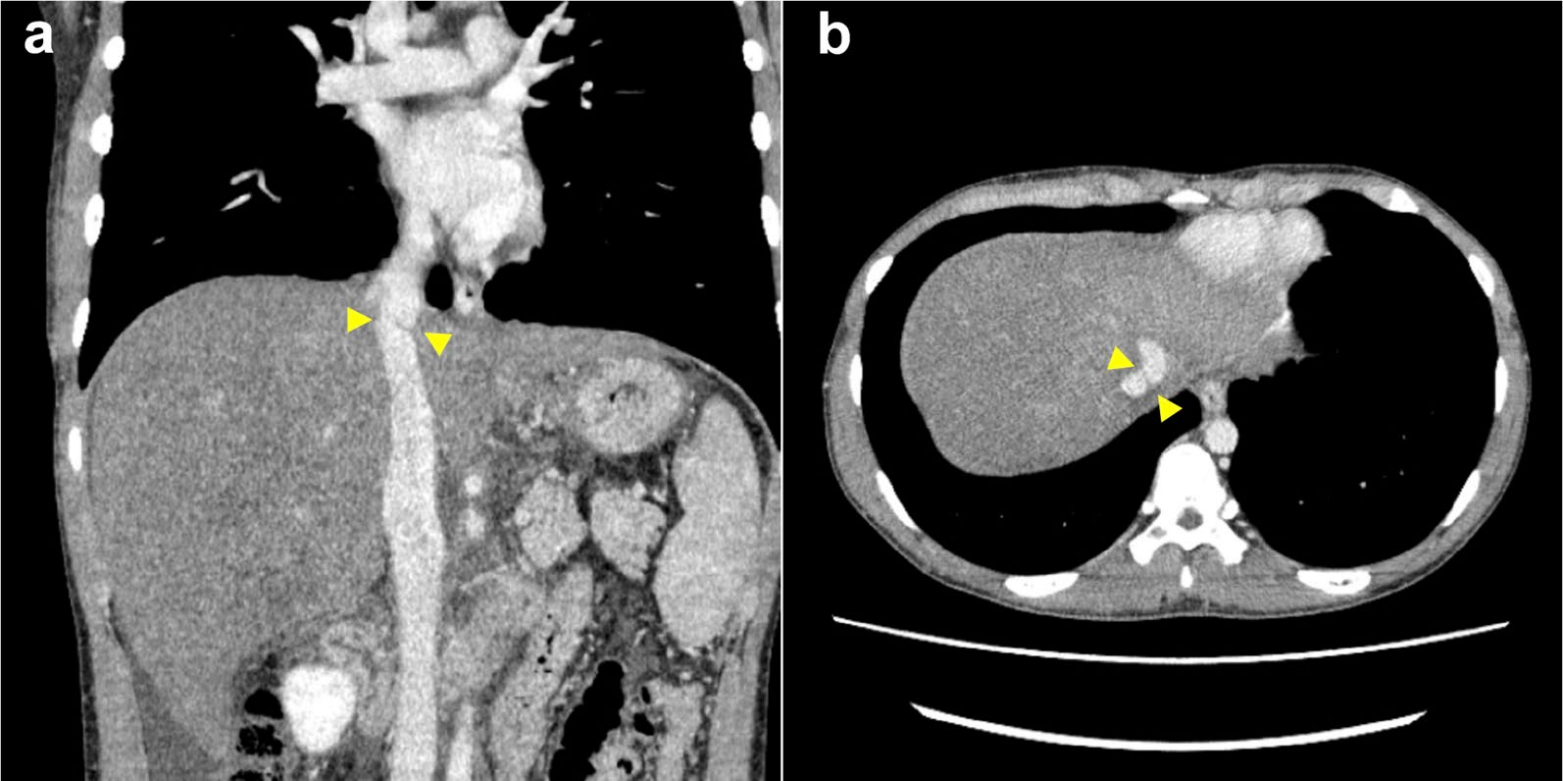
Preoperative abdominal computed tomography (CT) showing the membranous-like stenosis (yellow arrowhead) and non-perfusion of the main hepatic veins (a coronal section, b axial section)
